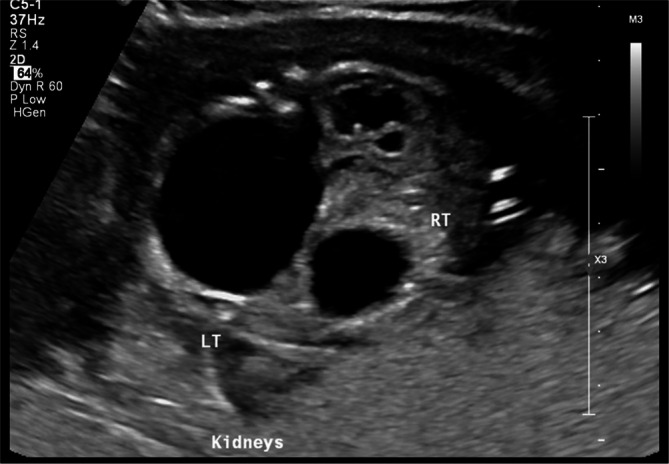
Prenatal ultrasound image in Case 3 at 19 weeks' gestation. Severely enlarged fetal bladder and bilateral hydronephrosis with mildly hyperechogenic kidneys and preserved corticomedullary differentiation. No cortical cysts are visible.
